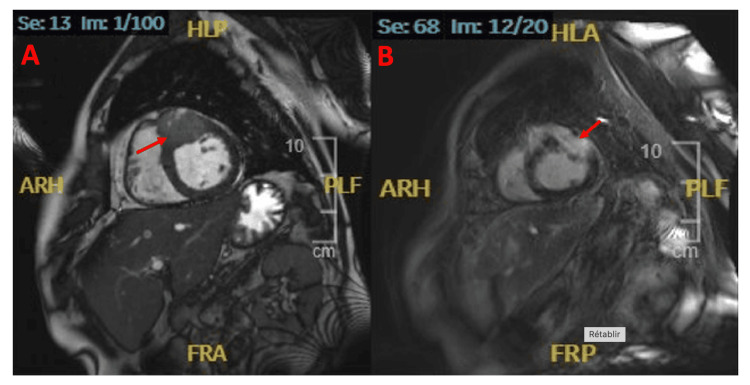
Cardiac MRI images showing the cardiac metastatic mass (red arrows) A: Short axis distal view. B: Short axis distal view with late gadolinium enhancement. Both views show the cardiac mass (measuring 25X20 mm involving the myocardium and located in the mid anteroseptal region) that was not seen in the short axis view from echocardiogram.
